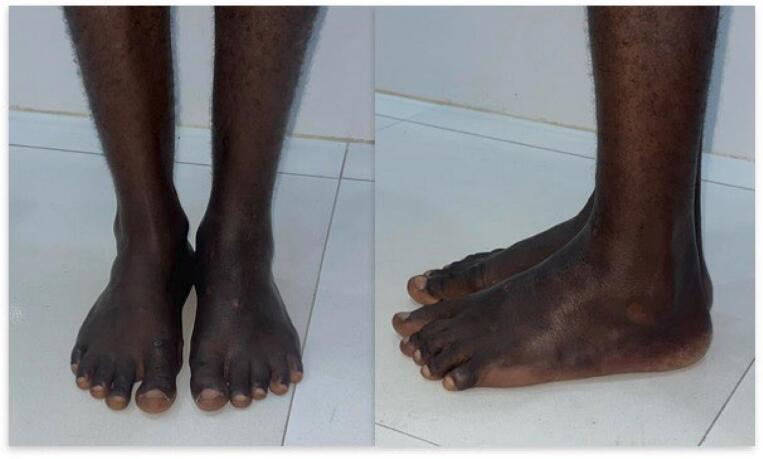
Frontal and lateral X-ray of the ankle 6 months after surgery.
